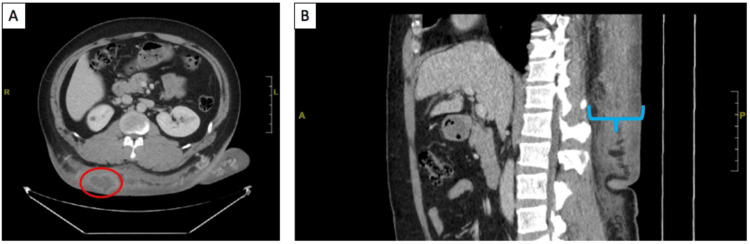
Abdominal CT. (A) Axial view. The red circle encloses the fluid collection measuring 5.0 x 3.2 x 4.0 cm. (B) Sagittal view of the patient. The blue bracket highlights the neurofibroma on the patient's back.
